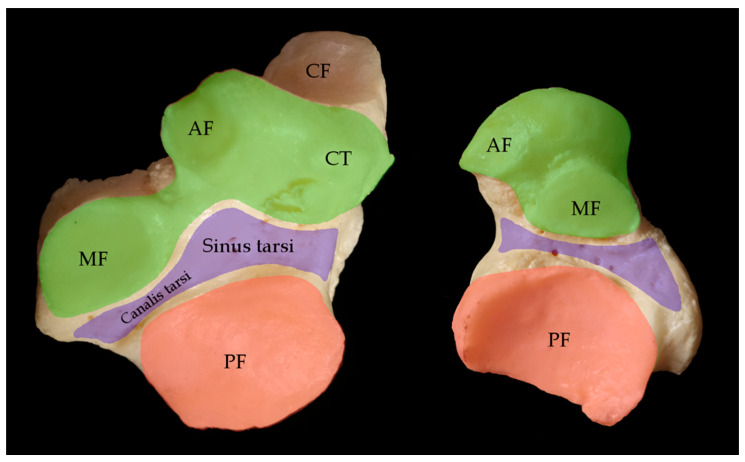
Compartments of the subtalar joint complex. Articular facets of the subtalar and calcaneonavicular joint complex of a right foot. Dorsal view of the calcaneus on the left, plantar view of the talus on the right site. The anterior compartment (green) consists of the anterior facet (AF), the middle facet (MF) and the crista lateralis (CT). The middle compartment (violet) is a conical tunnel, formed by the tarsal canal and tarsal sinus. The posterior compartment (red) consists of the posterior facet (PF). CF = cuboid facet.
